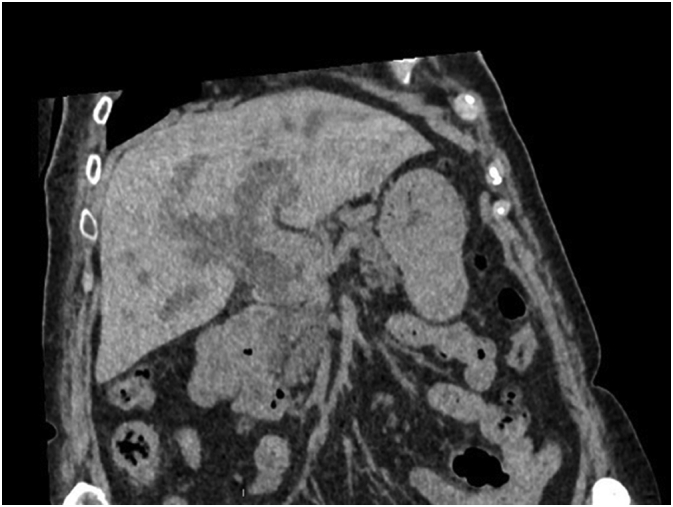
CT scan showing dilatation of intrahepatic biliary radicles with obstruction in the distal part of CBD.
